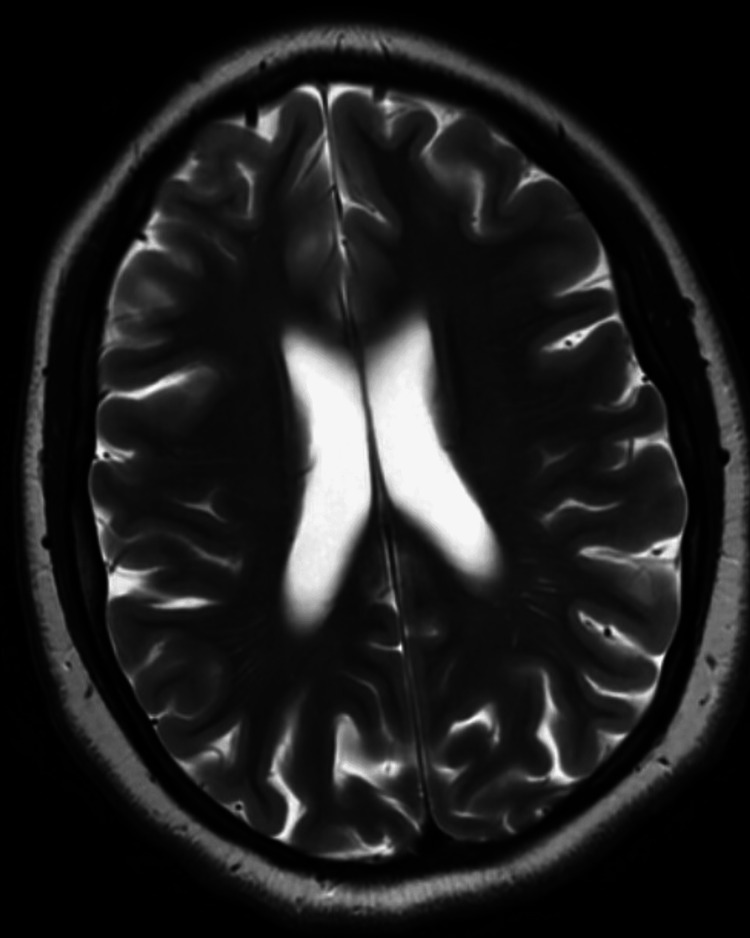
Abnormal cerebral atrophy. Magnetic resonance imaging (MRI) depicting generalized cerebral atrophy with the prominence of the sulci, fissures, and ventricles, abnormal for the patient’s age.
